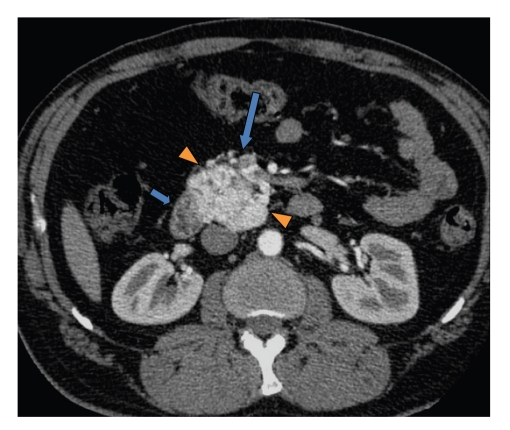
Axial contrast enhanced CT abdomen shows a hypervascular retroperitoneal mass (arrowheads) with enlarged surrounding vessels (large arrow). No cleavage plane is noted between the duodenum and the mass (short arrow). Also the mass contacts the inferior vena cava (IVC).
